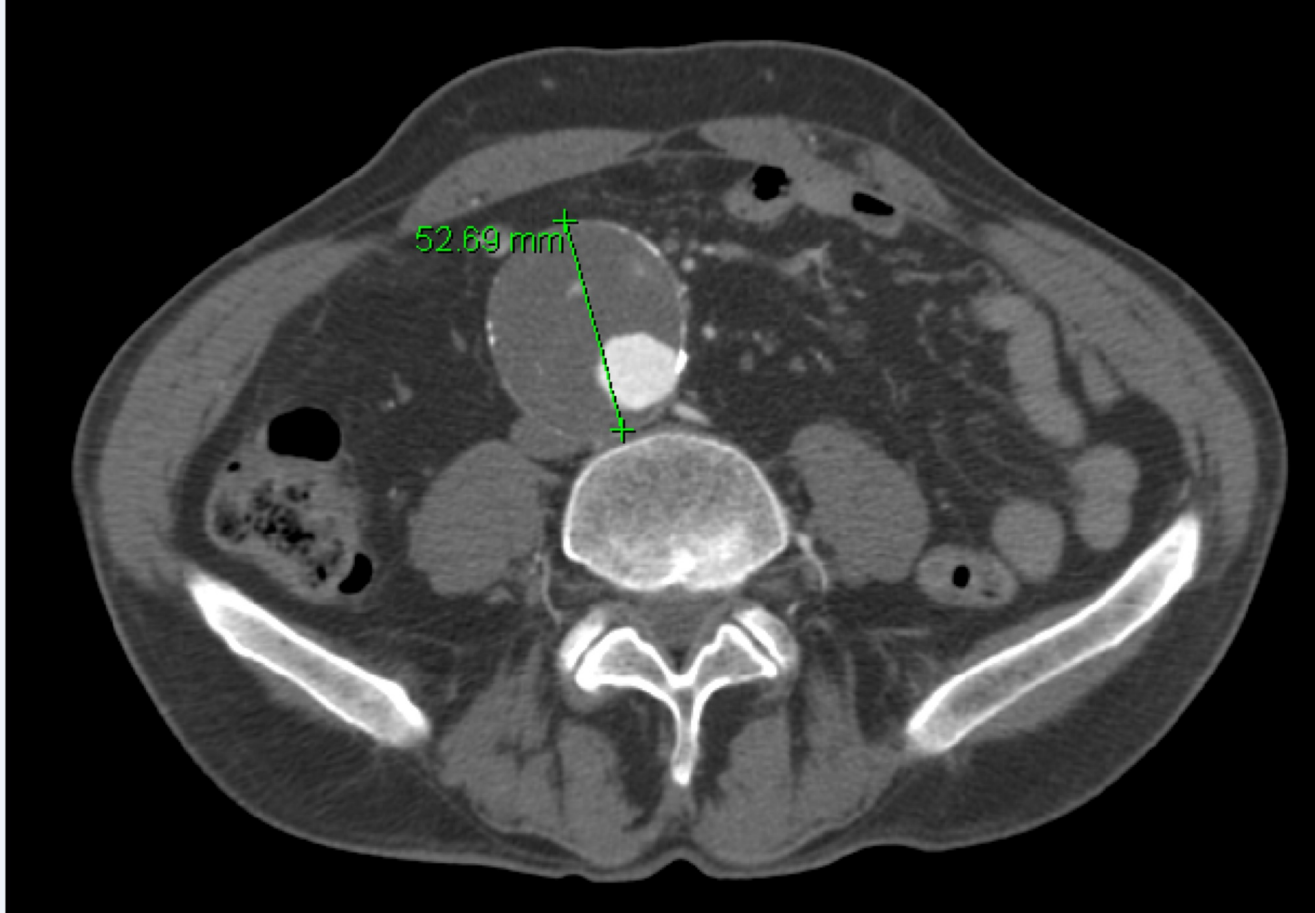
Preoperative abdominal CT angiography demonstrating AAA (green marker) AAA, abdominal aortic aneurysm
